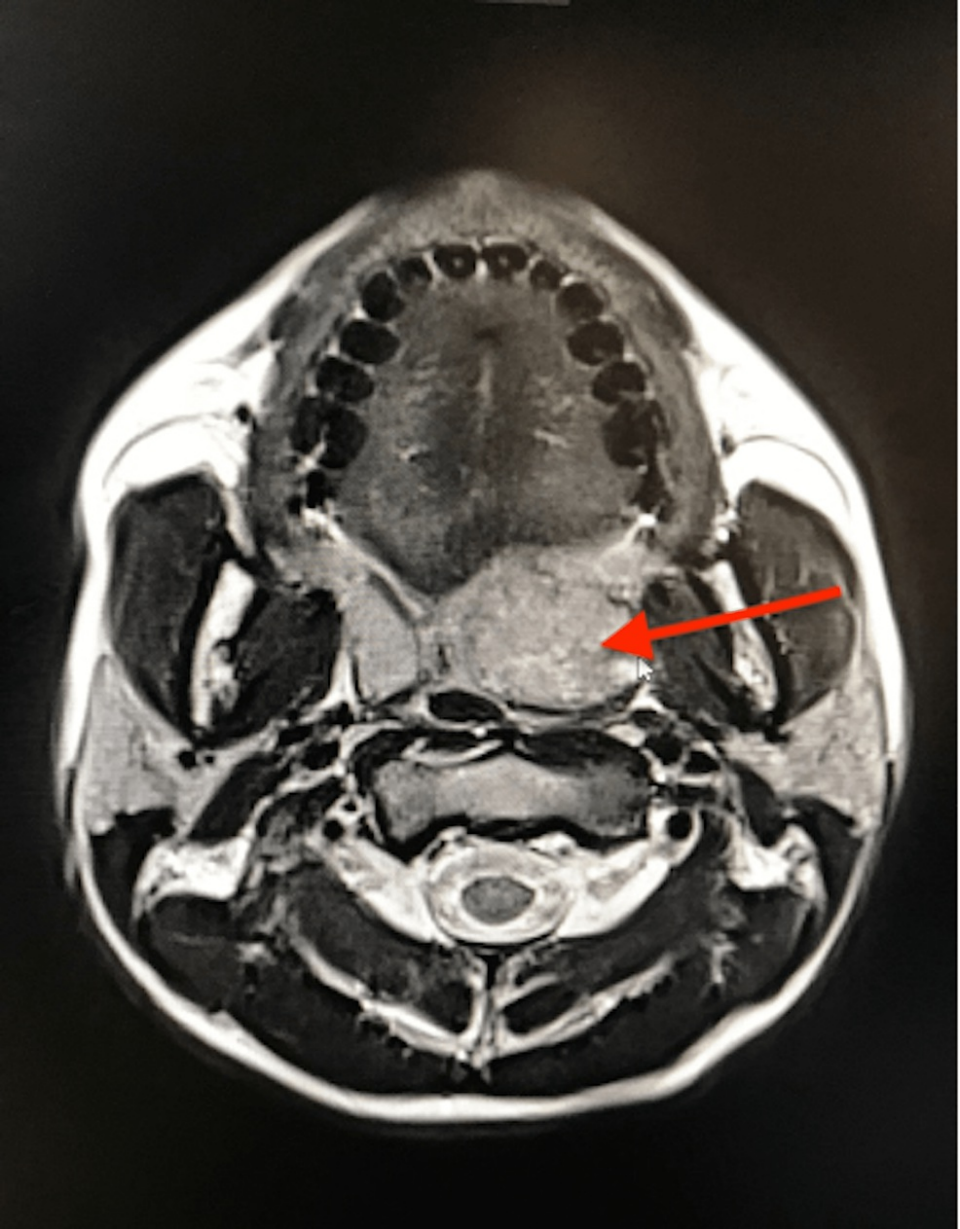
T1-weighted magnetic resonance imaging with contrast (axial section) showing a discrete heterogeneous contrast of the lesion (red arrow).
